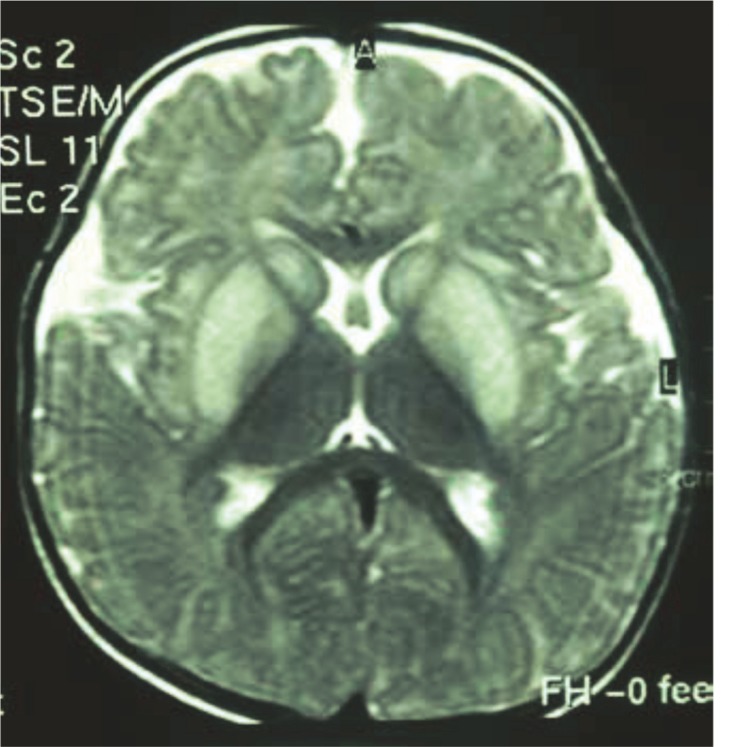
Abnormal Signal in Bilateral Basal Ganglia and Brain Atrophy in an MRI from a 4-year-old boy with propionic-acidemia
